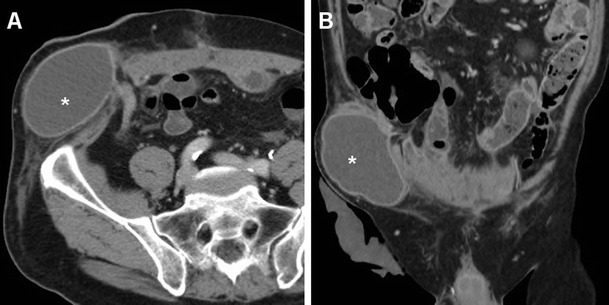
Failed repair of peristomal hernia in a 71-year-old male with a history of previous Bricker radical cystectomy, suffering from postoperative vomiting, persistent abdominal pain, and local swelling. Axial (a) and coronal (b) contrast-enhanced CT images showed recurrent herniation at the urostomy site of a dilated, fluid-filled structure consistent with the ileal conduit (*). Repeated surgery was performed
